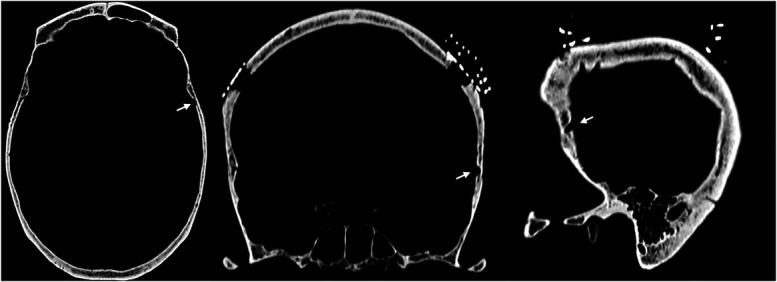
CT bone window of patient 4. The MMA sulcus (arrows) is clearly visible on all 3 planes. CT, computed tomography; MMA, middle meningeal artery.
